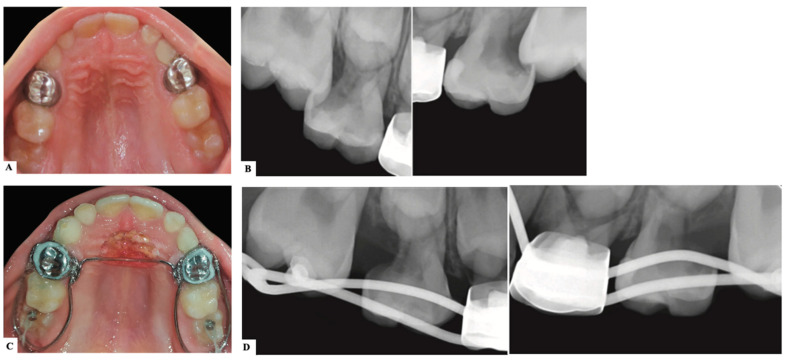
Bilateral ectopic maxillary first permanent molars with cemented modified Croll’s appliance to tip the first molars distally. (A). Pre-treatment clinical photograph showing bilateral ectopic eruption of the maxillary molars. (B). Pre-treatment radiograph. (C). Modified Croll’s appliance after cementation. (D). Post-treatment radiograph showing normal eruption of maxillary permanent molars. (Courtesy of Dr. Ambriss B, permission granted.)
